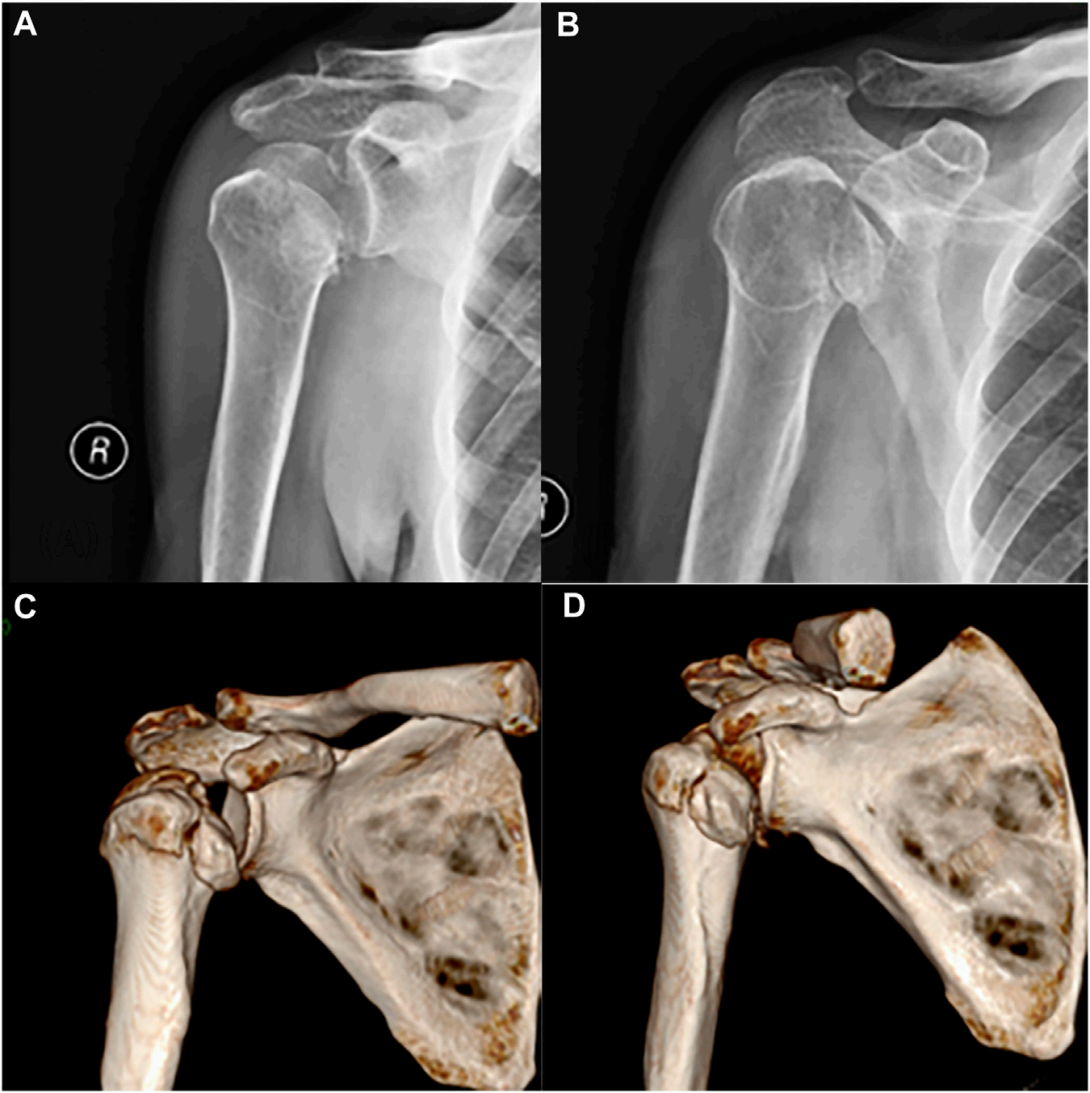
(A, B) Preoperative X-ray and (C, D) CT bone three-dimensional reconstruction.
